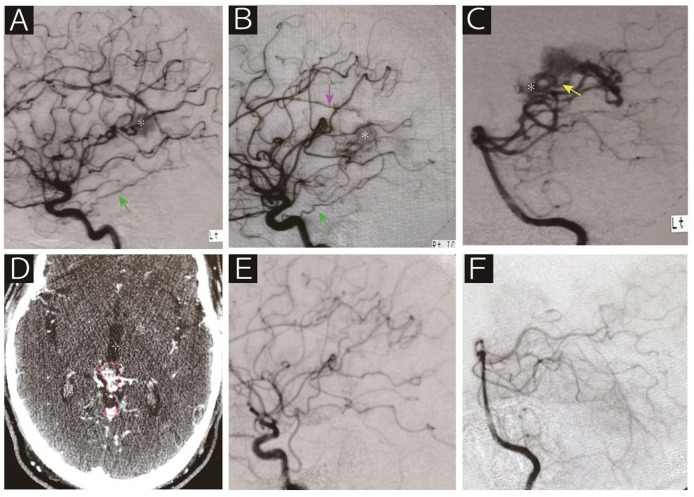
A 62-year-old man who presented with epilepsy was referred to us for radiosurgical treatment for an arteriovenous malformation. Digital subtraction angiography (A, left internal carotid artery; B, right internal carotid artery; C, left vertebral artery) showed that the nidus (asterisk) was supplied by the bilateral tentorial artery (green arrow), right callosal artery (purple arrow), medial posterior choroidal artery (yellow arrow) and posterior cerebral artery perforators. Contrasted computed tomography on the day of radiosurgery revealed the nidus adjacent to the vein of Galen (D). Note that the red line indicates the radiosurgical target. Angiographic obliteration was confirmed 40 months after radiosurgery without any deficits (E, right common carotid artery; F, left vertebral artery). The patient has been following a stable course over 10 years.
